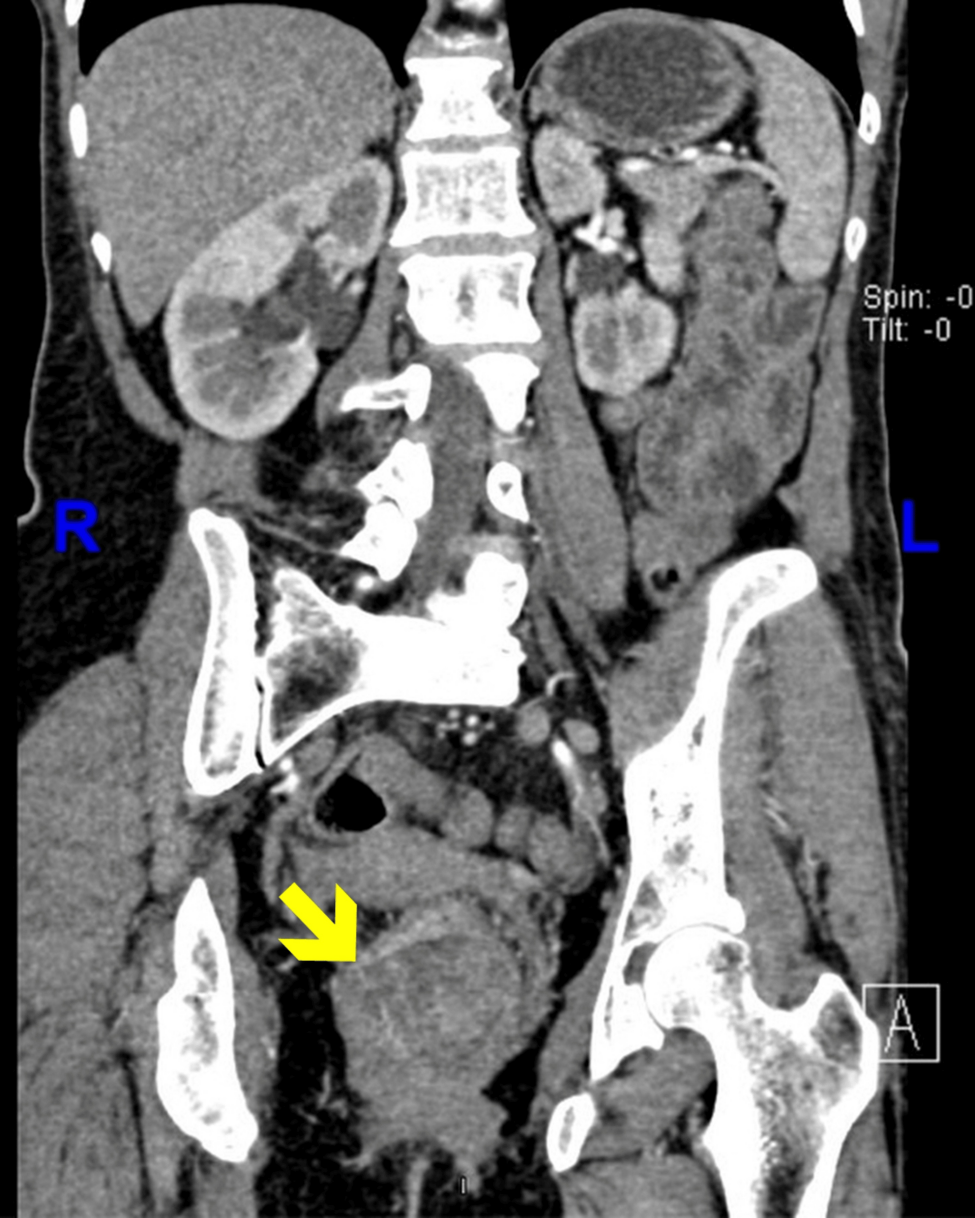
Coronal CT image (contrast-enhanced). Coronal contrast-enhanced CT scan of the abdomen and pelvis showing an irregular soft tissue mass within the lower pelvis, below the peritoneal reflection. The lesion displaces adjacent bowel loops and appears to distort the rectosigmoid contour. No radiographic evidence of hepatic metastases or retroperitoneal lymphadenopathy is observed.
